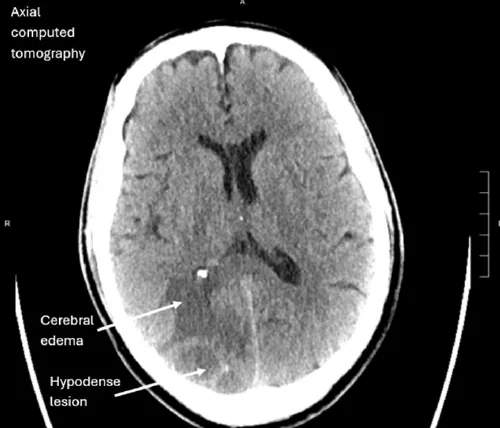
Axial computed tomography head image showing a 2.5 centimeter hypodense lesion in the right parietooccipital lobe with increased hypodensity in the right perirolandic region, concerning for cerebritis and possible intracranial abscess (white arrows).
